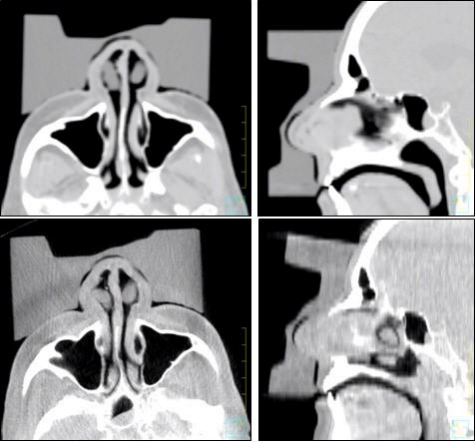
Axial and sagittal images of the reference plan CT (top) and a set of CBCT images from one of the treatment fractions (bottom). Notice the magnitude of tilt of the bolus in both the axial and sagittal CBCT images with respect to the planning images.
